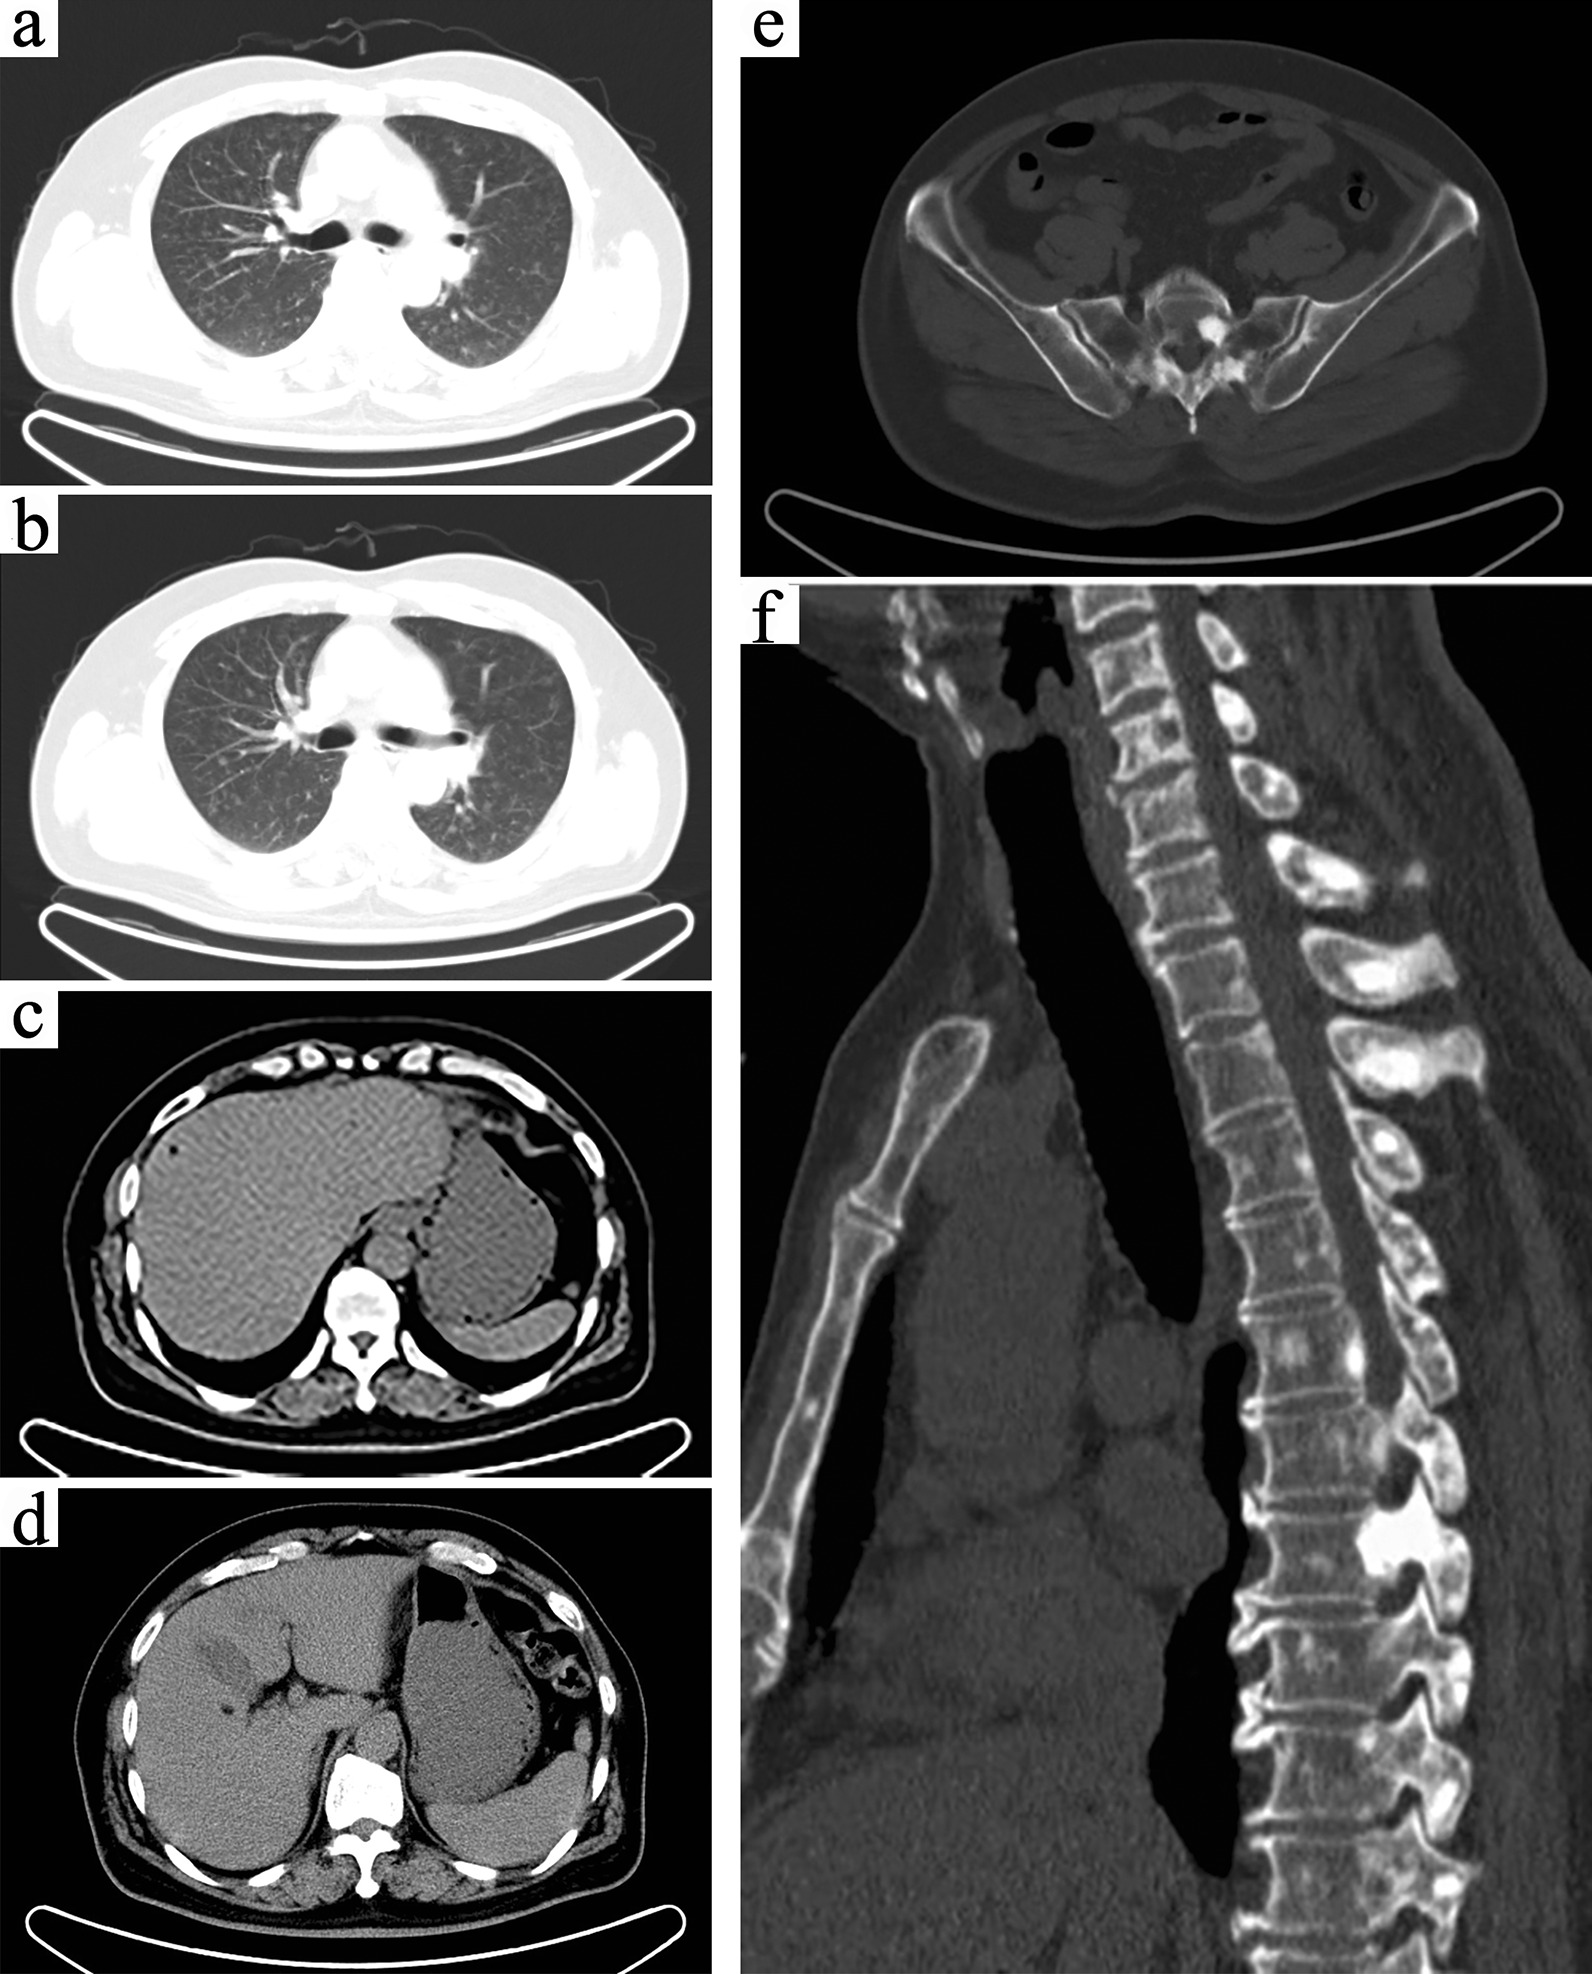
Images of the lungs, liver and bones in the PET-CT of the patient. a, b Diffuse ground glass density nodules throughout the lung fields. c, d Multiple low- density shadows containing fat density in the liver. e, f The bone mineral density of the thoracic vertebra and its appendages, sternum and bilateral ribs increased, showing hyperplastic and sclerosing changes
